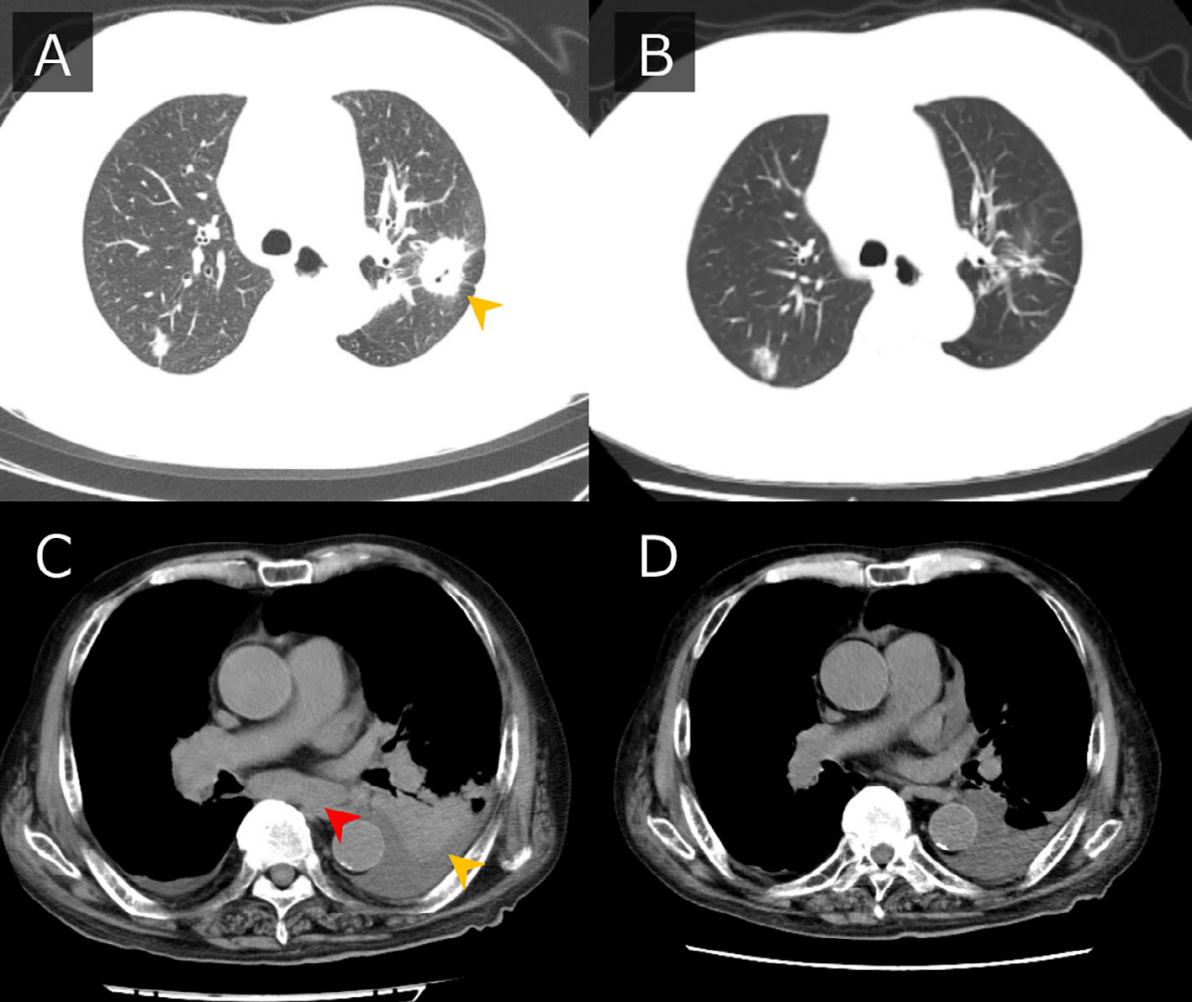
Computed tomography (CT) findings before the start of osimertinib treatment and at the time of the best response. In case 1, chest CT showed a mass (indicated by the yellow arrowhead) in the left upper lobe (A) that almost disappeared after three months of treatment (B). In case 2, chest CT showed a mass (indicated by the yellow arrowhead) in the left lower lobe and sub‐carinal lymph node metastasis (indicated by the red arrowhead) (C). After two months of treatment, the mass shrank and lymphadenopathy disappeared (D).
